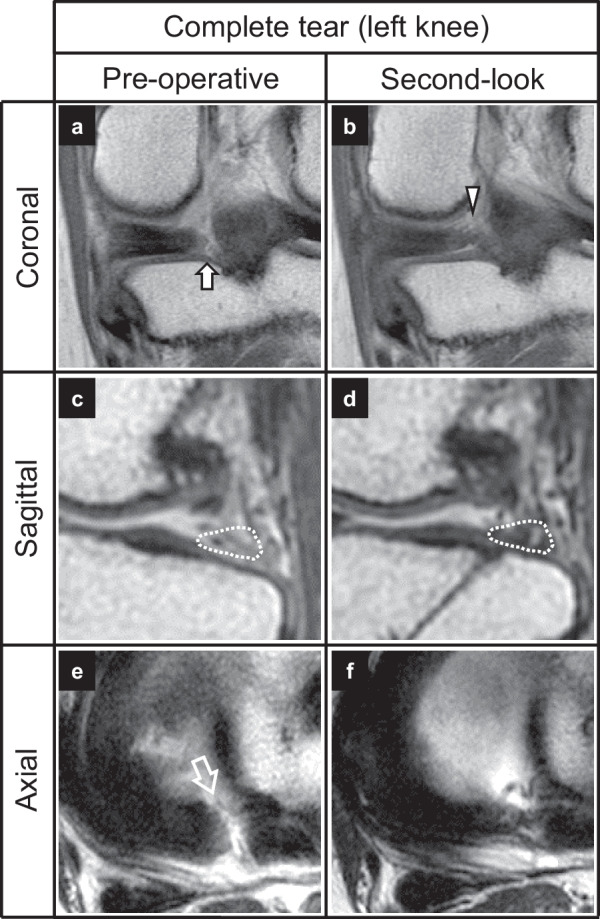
Pre- and postoperative MRI findings of a complete tear (same patient as in Fig. 3D–F). A Cleft sign (arrow). B No suspension bridge sign (arrowhead). C A ghost sign (dashed area). D Repaired root. E A radial tear sign (open arrow). F No radial tear sign. *MRI* magnetic resonance imaging
